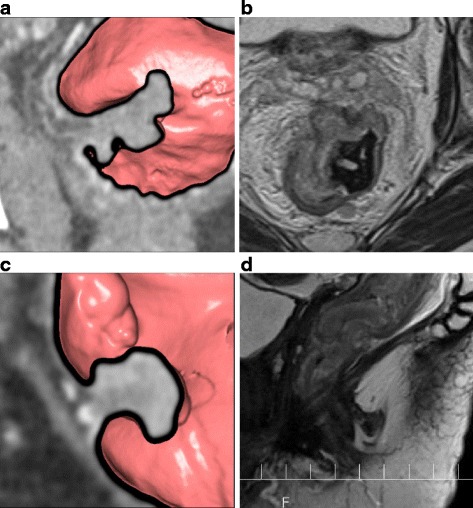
Two representative cases of pathological T2 tumors which were staged correctly by CTC with MPR but overstaged by MRI. (A, B): Patient in the seventies with pathological T2 lower rectal cancer, 45 mm in size. a Consecutive CTC imaging with MPR, aligned to the tumor axis, revealed a tumor with a smooth extramural layer, which was considered T2 tumor. b Axial T2-weighted MR imaging showed a tumor invading beyond the muscularis propria at right ventral side, which was considered T3 tumor. (C, D): Patient in the seventies with pathological T2 lower rectal cancer, 30 mm in size. c Consecutive CTC imaging with MPR, aligned to the tumor axis, revealed a tumor with a smooth extramural layer, which was considered T2 tumor. d Sagittal T2-weighted MR imaging showed a tumor invading beyond the muscularis propria at left dorsal side, which was considered T3 tumor
